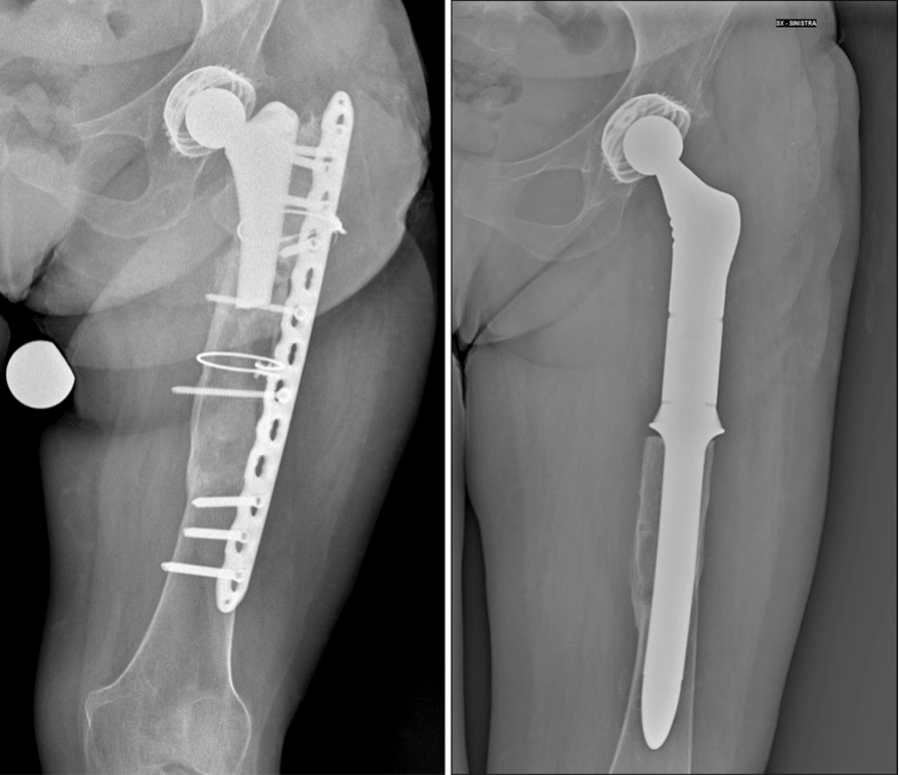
Pre- and postoperative radiographies of a patient with periprosthetic and prosthesis component fracture treated first with plate and cable fixation and then, because of persistent pain and unstable fixation, with proximal femoral replacement
